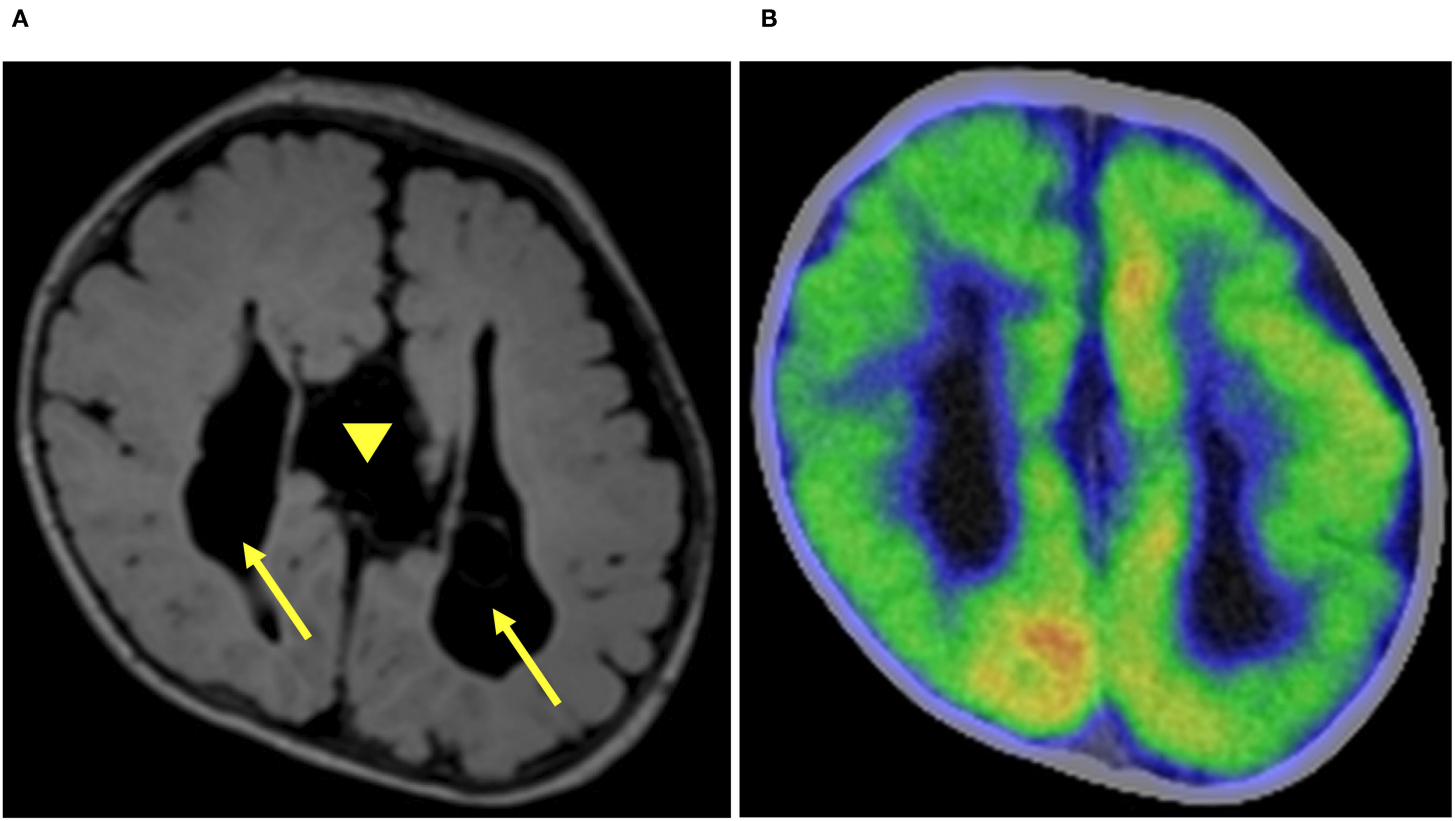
Preoperative imaging. Preoperative axial fluid-attenuated inversion recovery magnetic resonance imaging (FLAIR MRI) demonstrated corpus callosum agenesis (arrow head) and bilateral (right dominant) ventricular dilatation (arrow) (A). Fluorodeoxyglucose-positron emission tomography-computed tomography showed hypometabolism in the right hemisphere (B).
